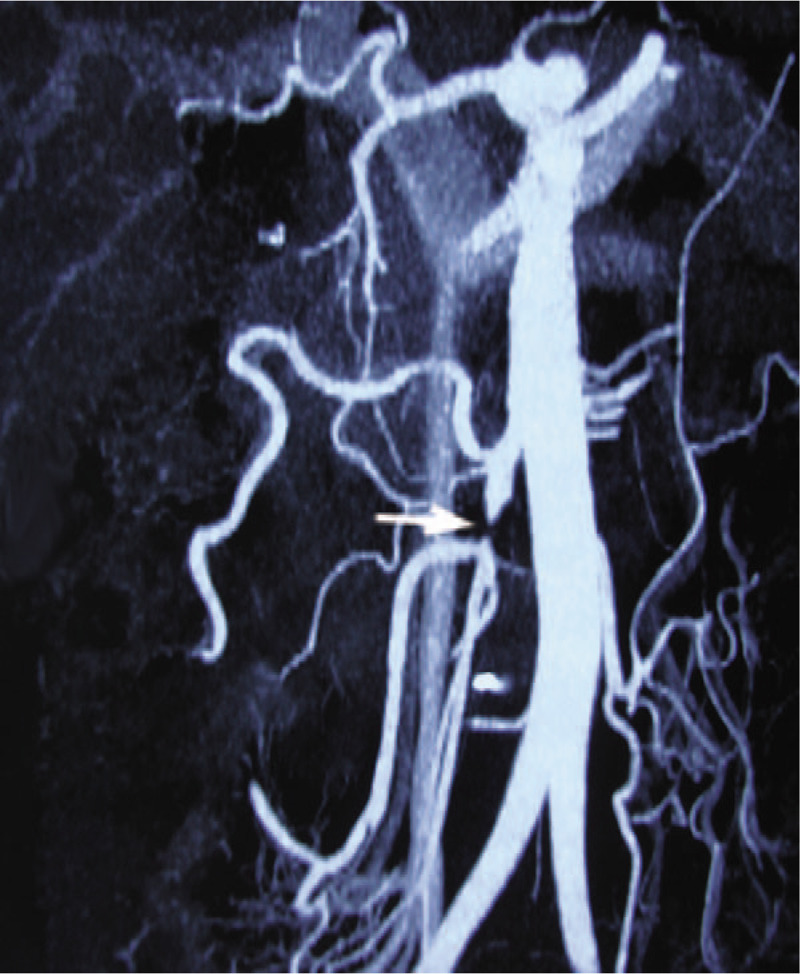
One month after the operation, computed tomography angiography (CTA) showed the main superior mesenteric artery (SMA) trunk remained occluded for about 1 cm (white arrow), but the collateral pathway can supply the small intestinal branches of the superior mesenteric artery.
